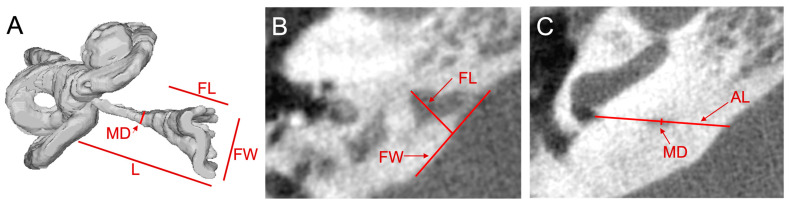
Cochlear aqueduct measurements of right ear on flat-panel CT scan. (A) 3D reconstruction of an inner ear showing the location of measurements of the cochlear aqueduct (CA) recorded from the axial slices shown in *B* and *C*. Midpoint diameter (MD), length (L), and medial aperture funnel length (FL) and funnel width (FW) are labeled. (B) Axial slice at the level of the greatest funnel width labeled with FL and FW which are measured in this image. (C) Axial slice at the level of the CA’s opening into the inner ear near the basal turn of the cochlea; MD and axial length (AL), or length of CA in the axial slice, are labeled.
